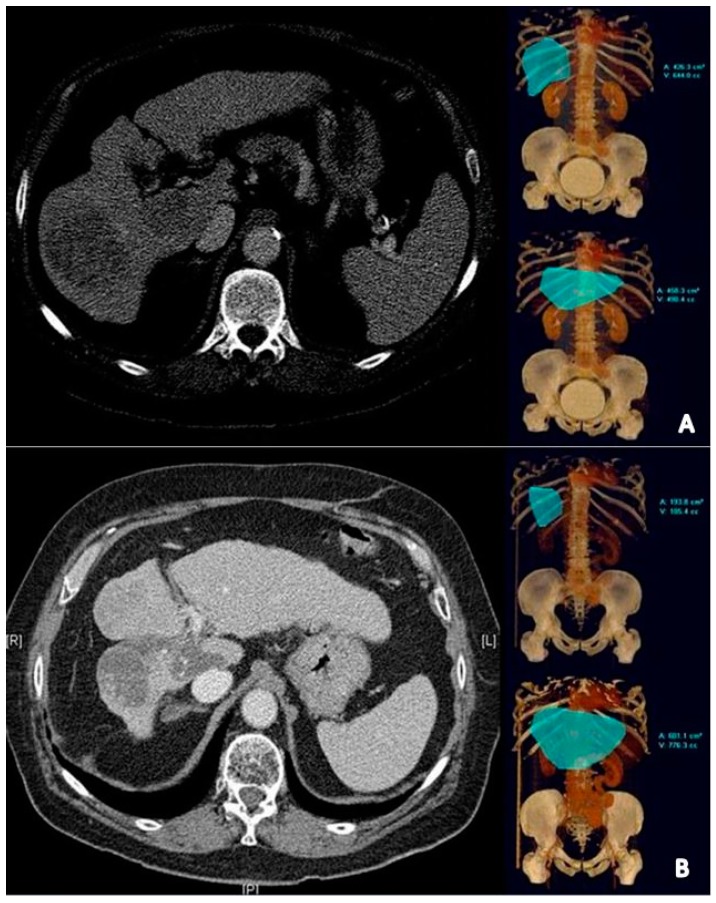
Pre- and post-treatment radiation lobectomy imaging. (A) Pre-treatment arterial phase contrast-enhanced CT showing multifocal right lobe HCC. Pre-treatment right and left HLVs are 644 and 498 mL, respectively. (B) Post-treatment contrast-enhanced CT demonstrates right lobe atrophy, tumor necrosis, and left lobe hypertrophy. Post-treatment HLVs are 185 mL and 776 mL, respectively. Reproduced with permission from [49].
